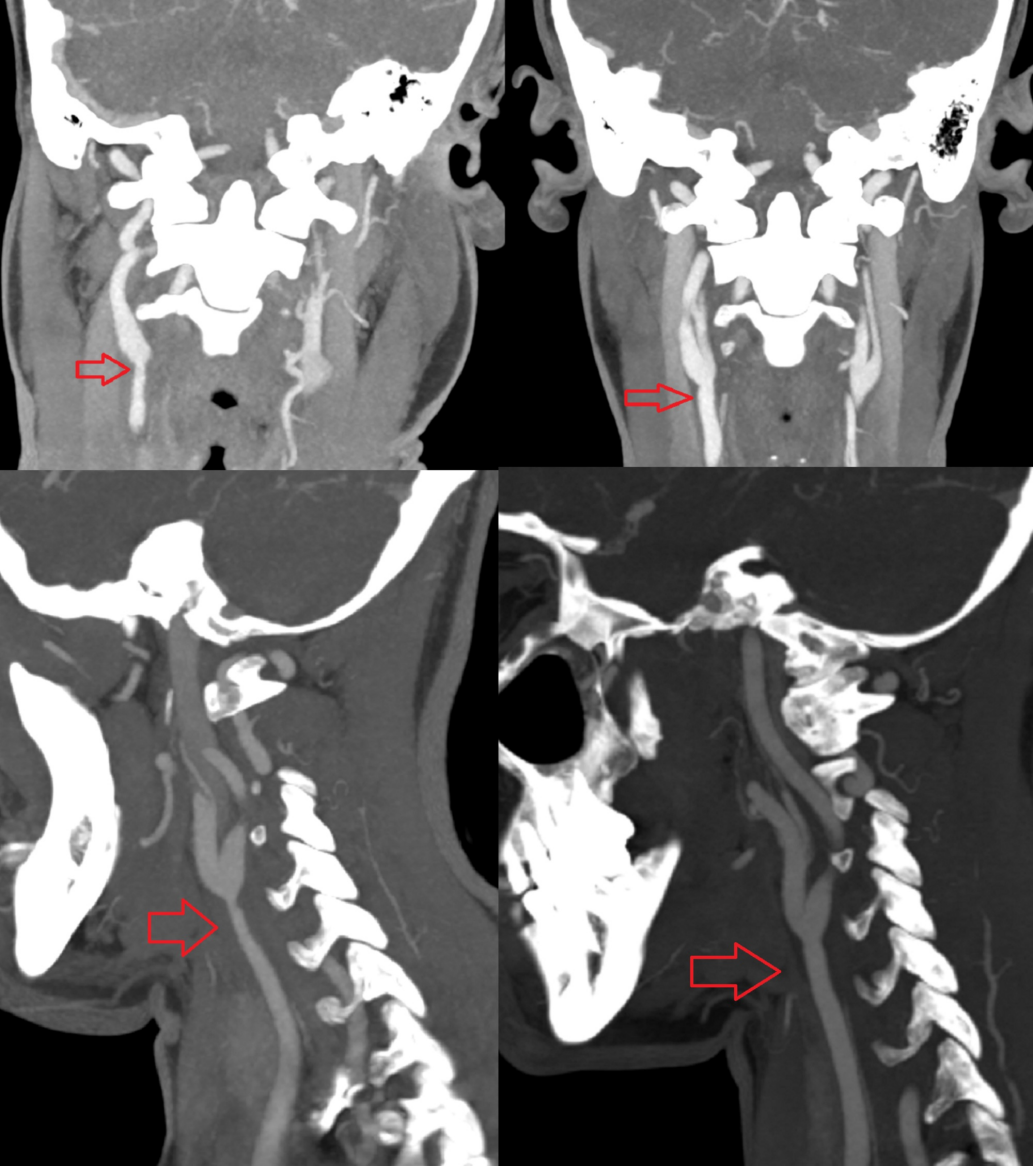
CT angiography of the neck vessels Right common carotid artery stenosis (right upper and maximum intensity projection [MIP] images on right lower) at the initial presentation and improvement of the stenosis after treatment on follow up images (left upper and MIP images on left lower) in three months.
